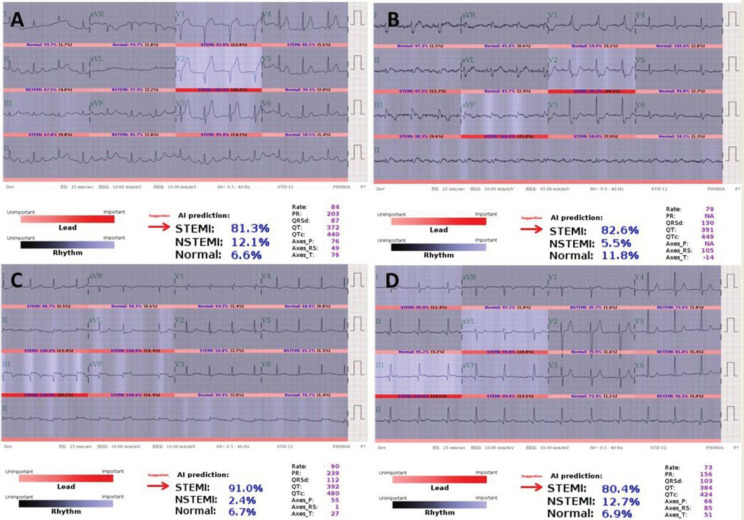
STEMIs for which alarms were raised by the AI-based alarm strategy but for which diagnosis by front-line physicians were delayed. (A) A 36-year-old tall man awaiting chest X-ray to rule out pneumothorax. (B) An 82-year-old woman presenting with left shoulder pain initially. (C) A 75-year-old woman presenting with acute epigastric pain, nausea, and dizziness awaiting an X-ray to rule out a perforated peptic ulcer. (D) A 54-year-old man with a chronic kidney disease awaiting labs to rule out hyperkalemia.
